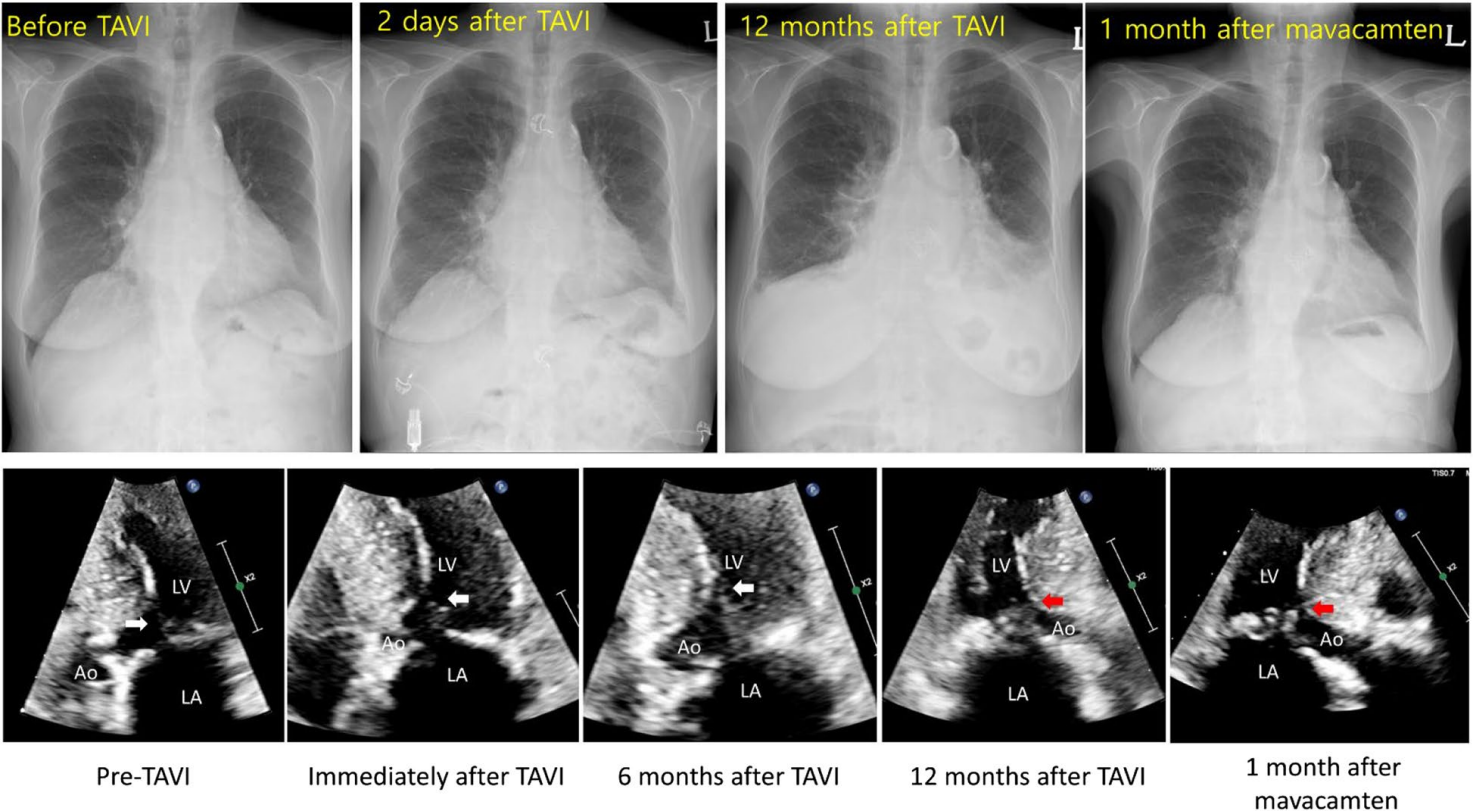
A representative case showing the development of progressive heart failure after uneventful transcatheter aortic valve implantation. An 87-year-old woman developed progressive dyspnea 12 months after uneventful transcatheter aortic valve implantation. A Chest x-ray showed pulmonary edema and bilateral pleural effusion. B Echocardiography showed progressive development of prominent systolic anterior motion of the mitral valve resulting in septal contact (arrows). A cardiac myosin inhibitor dramatically decreased SAM-septal contact, with improvement of symptoms and chest x-ray findings. Reproduced from Jo et al. [55], available under the Creative Commons license
